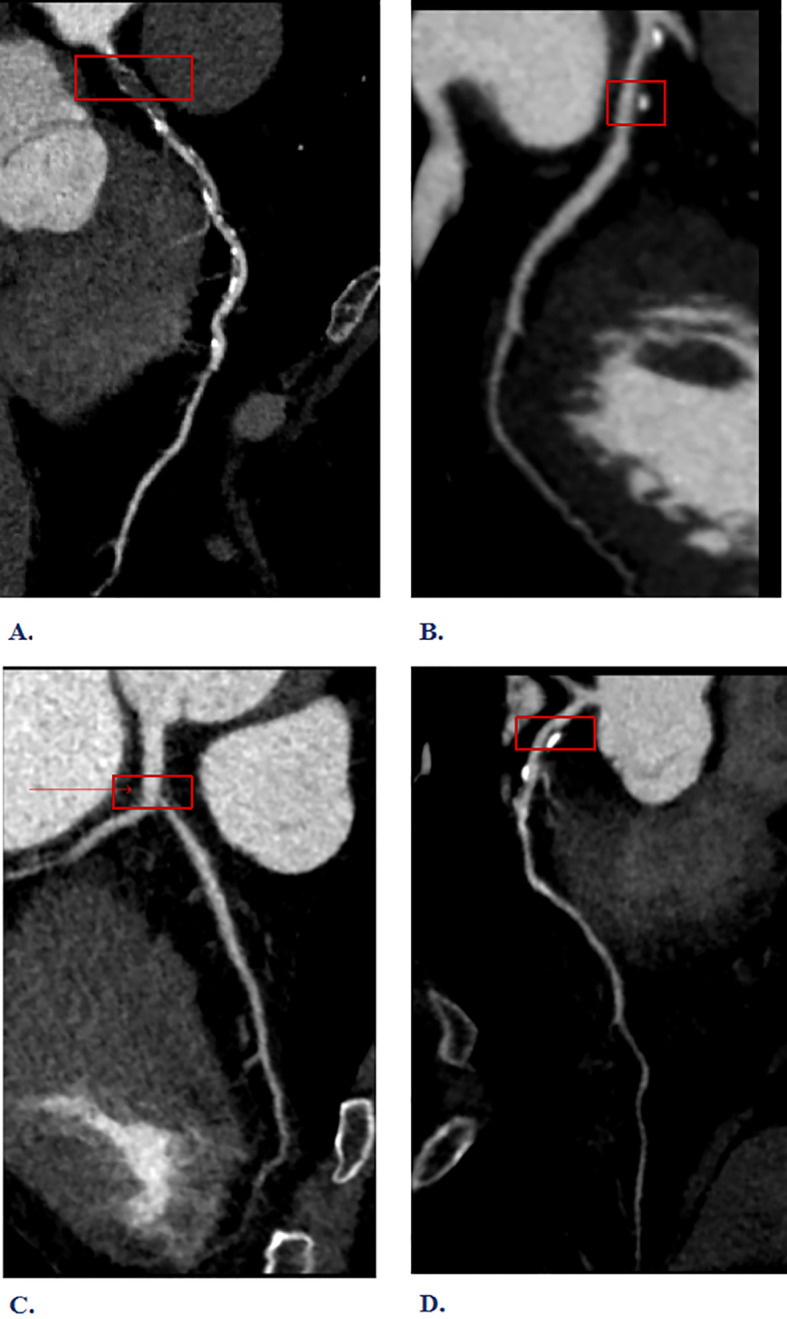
Interpretation of high-risk plaque results from Coronary Computed Tomography Angiography (CCTA). (A) Napkin-ring sign (B) Spotty calcification (C) Low-density plaques (D) Positive remodeling.
